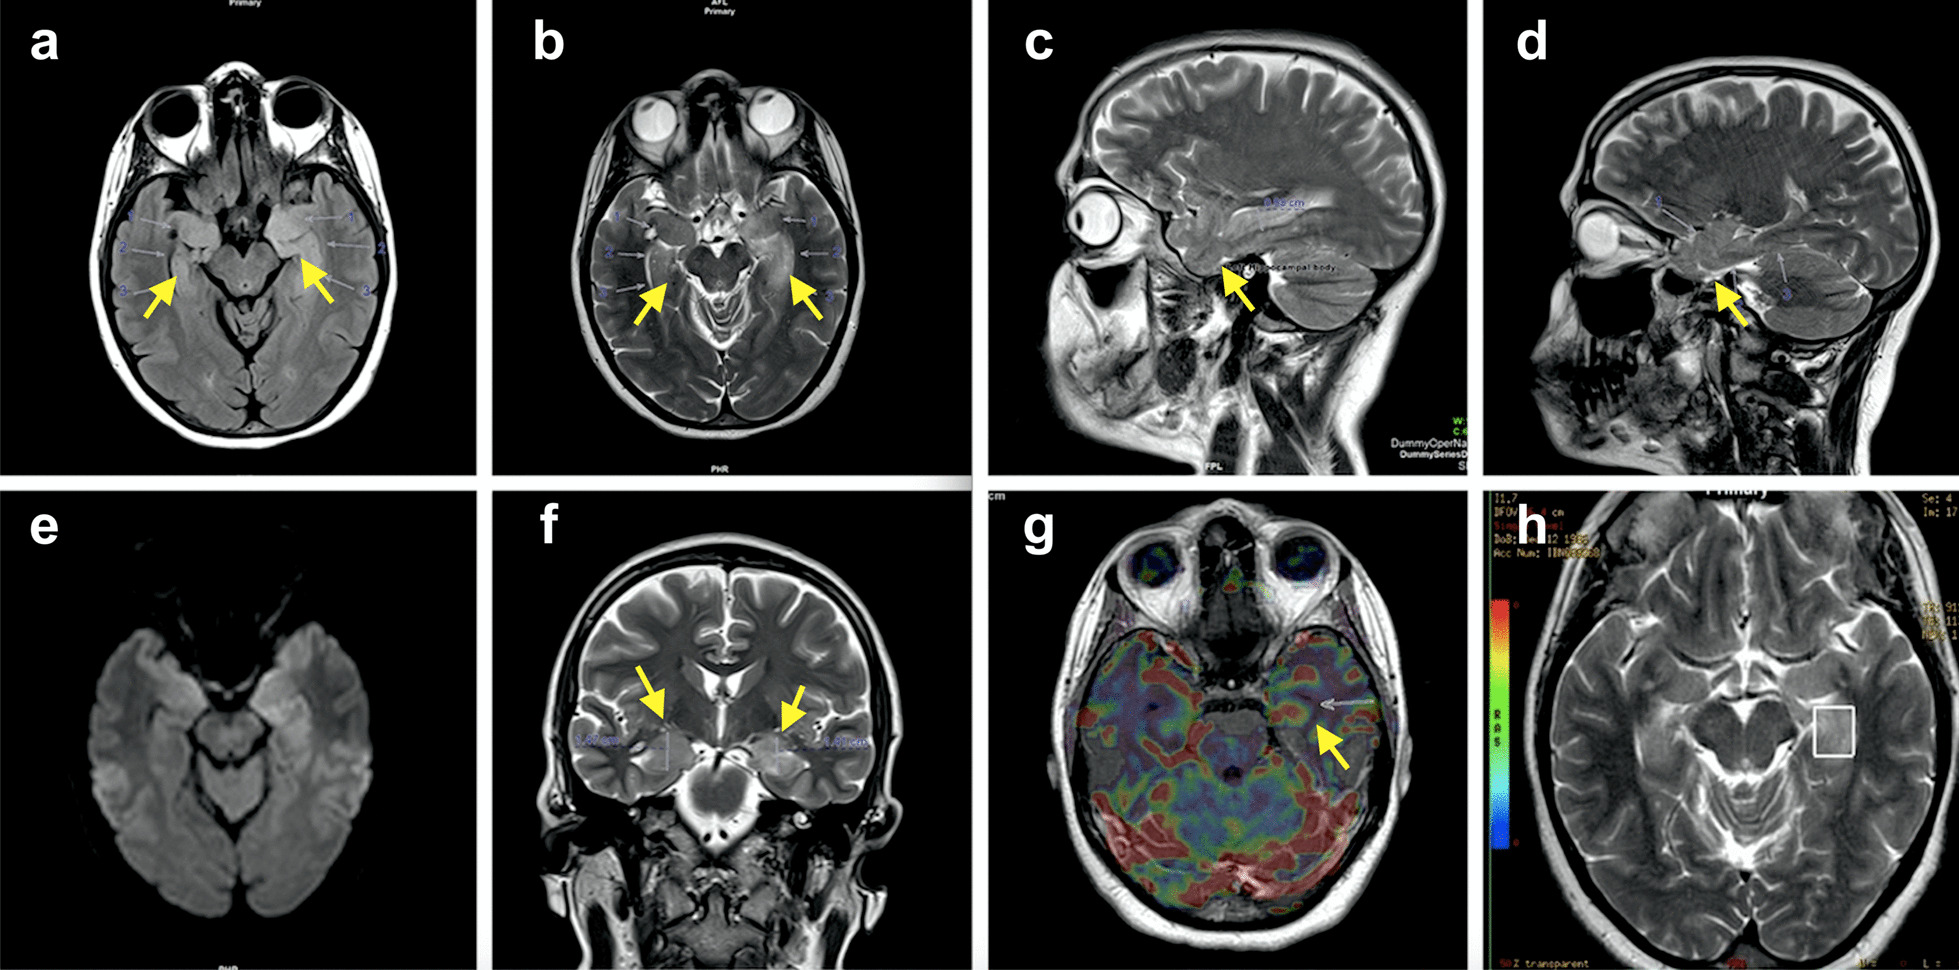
Magnetic resonance imaging findings. a Axial fluid-attenuated inversion recovery (FLAIR), b axial T2-weighted images, showing bilateral hyperintense
